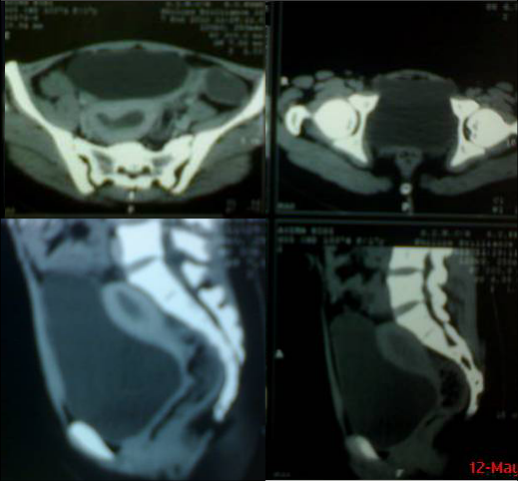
Figure 1:CT scan showing dilated uterus in upper right film. Lower vertical sections showing bladder with adjacent rectum inferiorly and uterus superiorly. No vaginal tissue below uterus is apparent.
